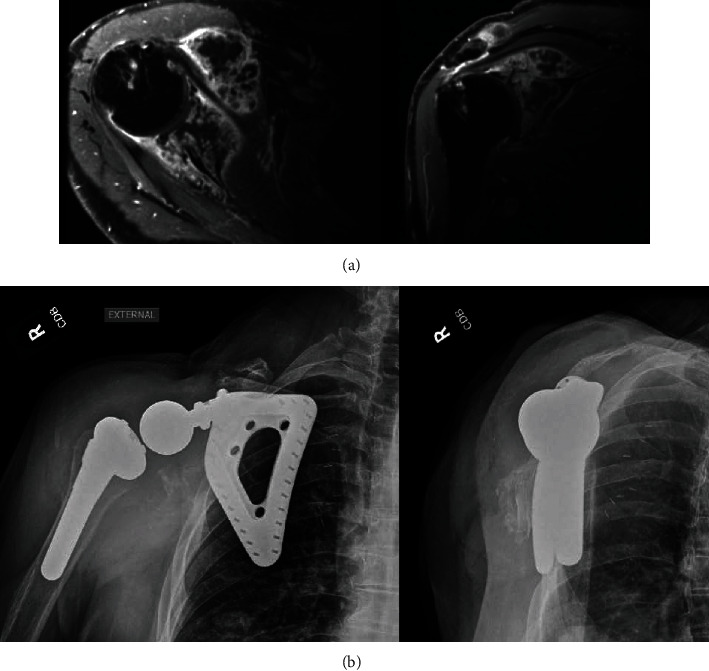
(a) MRI demonstrating expansive, infiltrative soft tissue malignancy about the scapula and shoulder girdle. (b) Eight-month postoperative radiographs demonstrating well-fixed implants in appropriate alignment.
